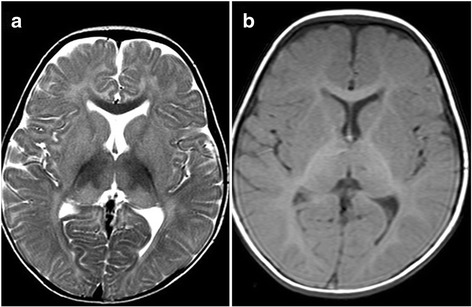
(a)-(b): Initial T2 weighted MRI pictures of brain revealed (a) putaminal hyperintensity and (b) thalamic hypointensity with some unmyelinated white matter in T2/T1 weighted images
